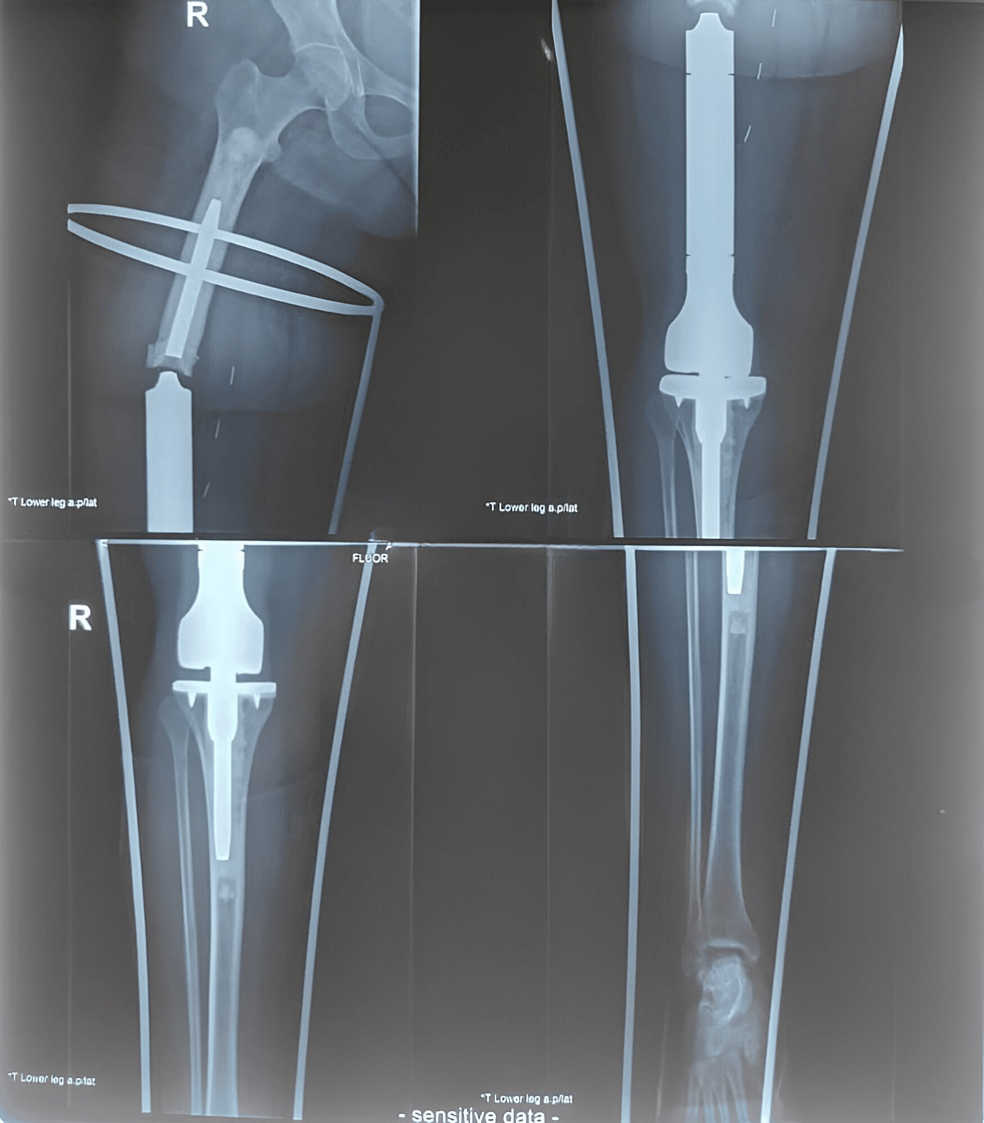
X-ray showing the broken implant of the megaprosthesis
